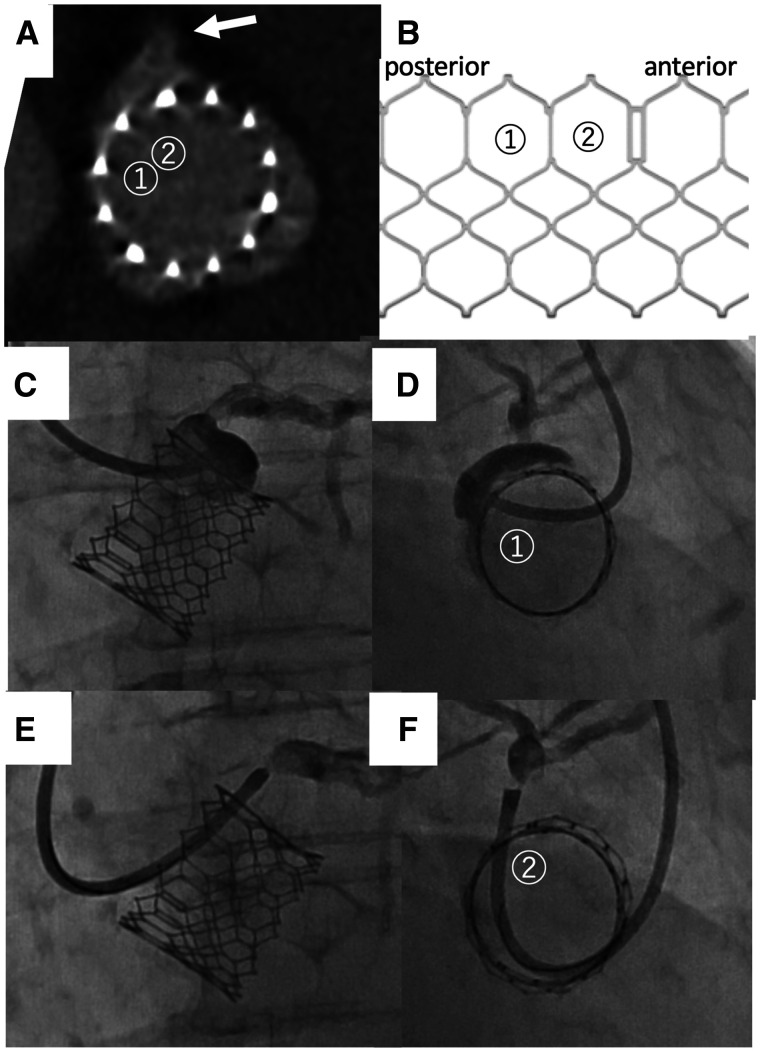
Patient 1: Appropriate selection of transcatheter heart valve cells under guidance of the en face view using the deep right-anterior-oblique cranial position (right-anterior-oblique 71°, cranial 35°) to achieve selective coronary engagement. (*A*) Short-axis computed tomography image after transcatheter aortic valve implantation. The arrow indicates the left main coronary artery. (*B*) The SAPIEN 3 frame. A 6-Fr ASAHI Hyperion Super Power Backup 3.0 catheter was selected as the guide catheter from the left radial artery. ① indicates the cell crossed in (*C* and *D*). ② indicates the cell crossed in (*E* and *F*). On the first attempt, the guide catheter was crossed through cell ①. Angiograms taken from the perpendicular (*C*) and the en face views (*D*) simultaneously. The en face view (*D*) shows the guide catheter passing through the cell posterior to the left main coronary artery. On the second attempt, the catheter was passed through cell ② in front of the LMCA ostia (*E* and *F*).
